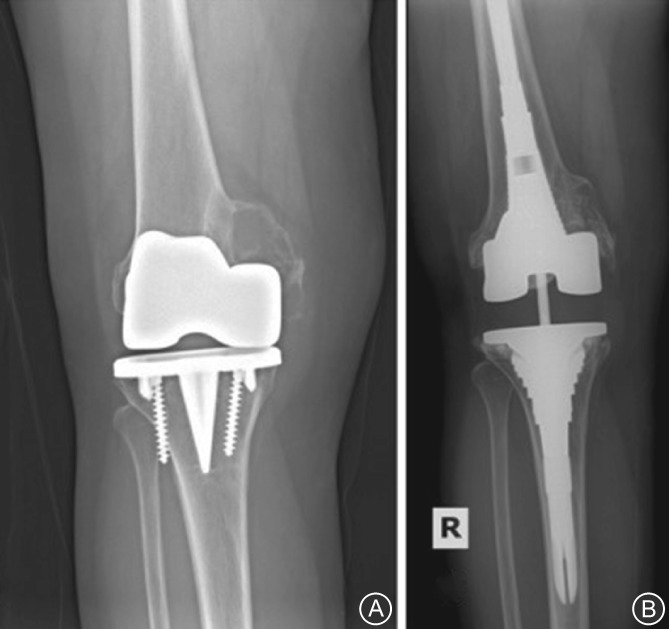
(A) Preoperative anteroposterior (AP) radiograph showing a Type IIA Anderson Orthopedic Research Institute tibial defect. (B) Postoperative AP radiograph (33 months) showing a cementless metaphyseal sleeve and stem construct.
